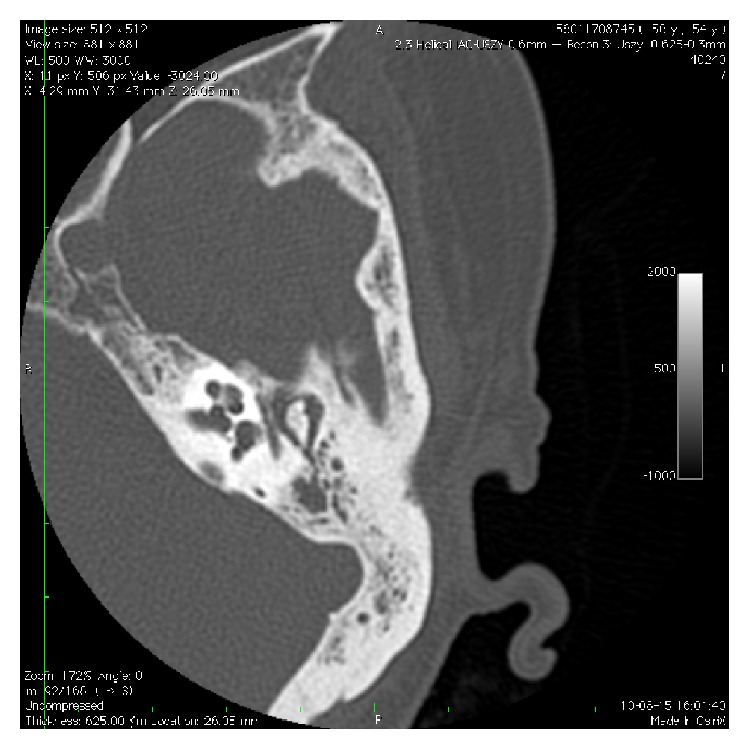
CT scan shows sclerotic left mastoid process and oppacified tympanic cavity.
